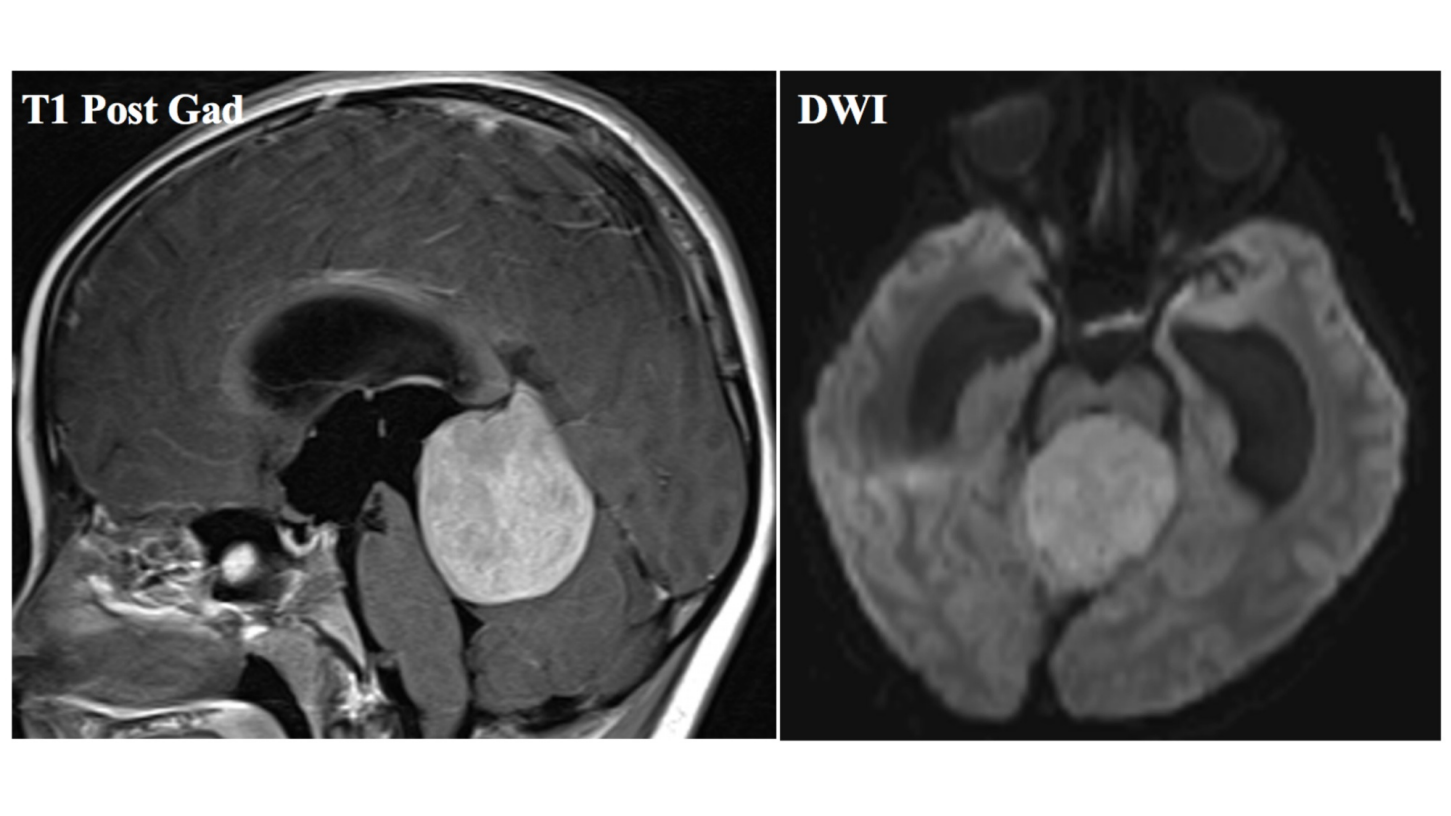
High-grade pineal parenchymal tumor at presentation Demonstrates an avidly enhancing mass involving the pineal gland with mass effect on the cranial portion of the vermis. The mass demonstrates evidence of reduced diffusion on DWI, suggesting increased cellularity within the tumor. DWI: diffusion-weighted imaging
